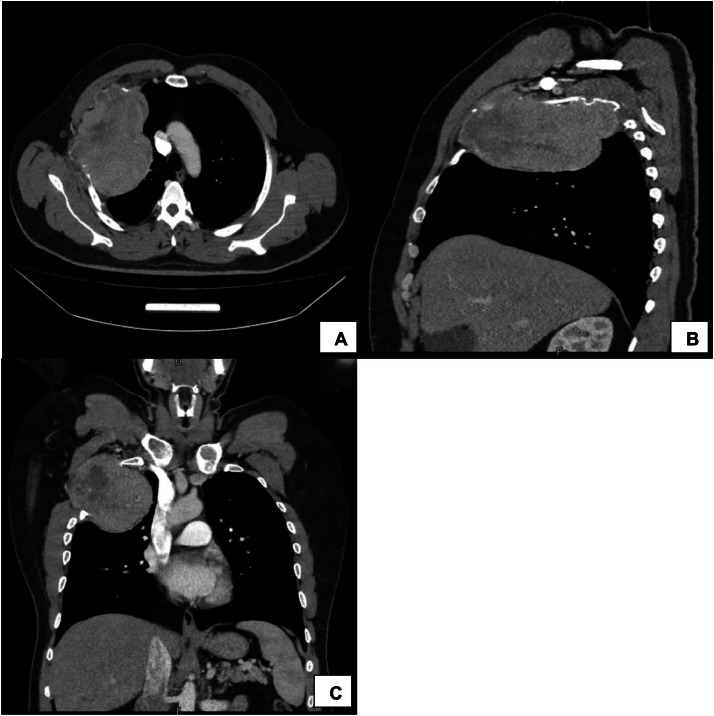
A: Axial B: Sagittal C: Coronal CT scan with contrast of the thorax showing a homogeneously enhancing soft tissue mass measuring 14 × 11 cm in the right upper anterior chest wall with pathological destruction of the right second rib.
